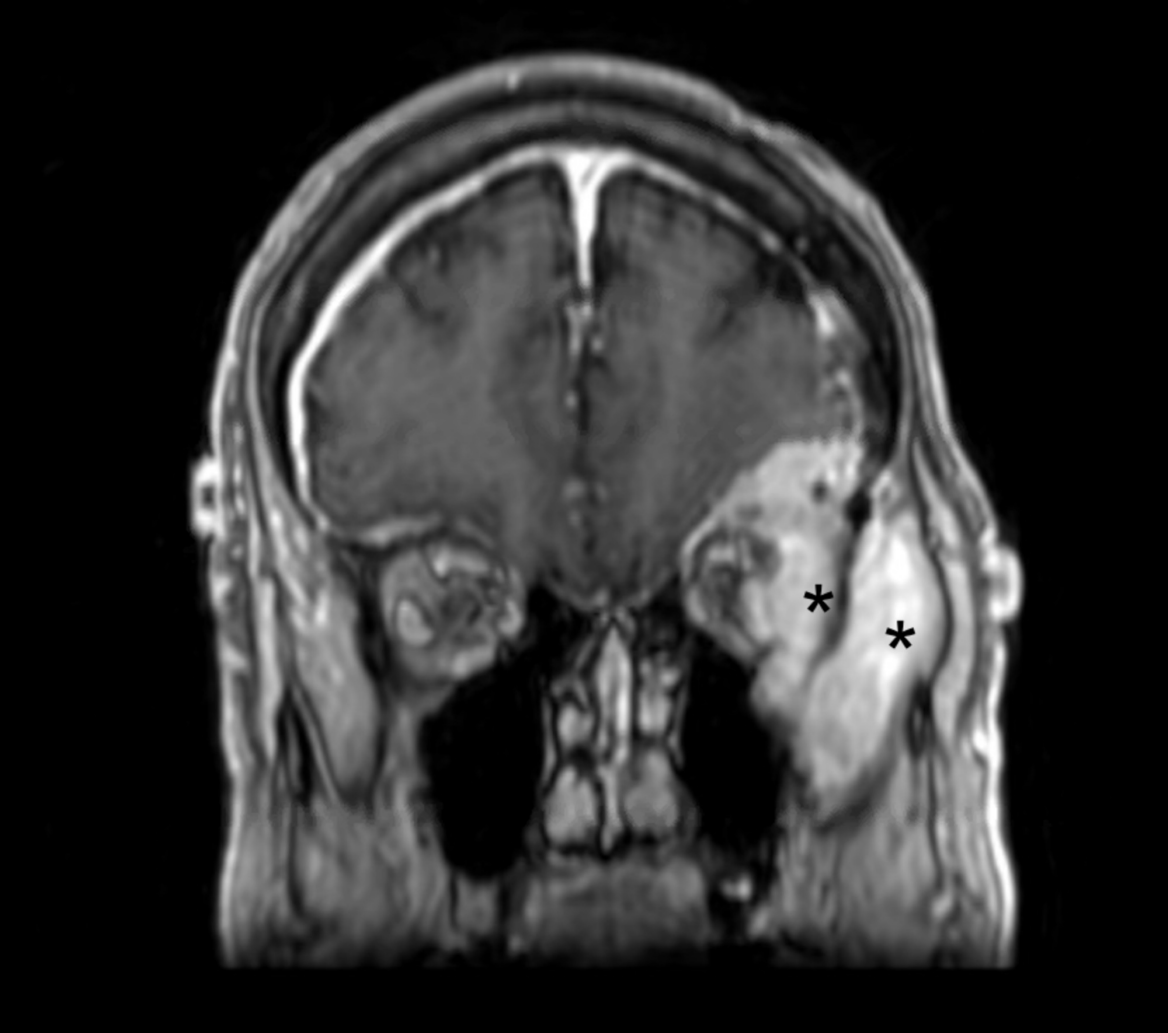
Preoperative coronal MRI demonstrating tumor involvement of the lateral orbit and temporalis muscle * = Tumor invasion of the lateral orbit and temporalis muscle MRI: magnetic resonance imaging
